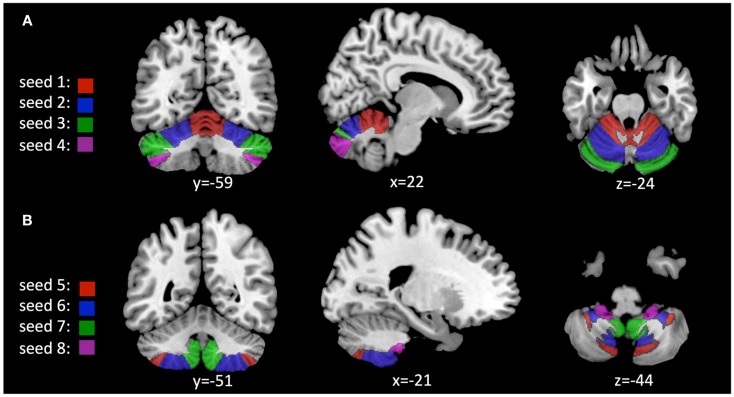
Location of seeds used for analysis of cerebro-cerebellar coupling as derived from the AAL 3D MRI atlas (Schmahmann et al., 1999; Tzourio-Mazoyer et al., 2002): (A) Seeds #1–4, representing cerebellar lobules I–V; VI; crus 1; crus 2. (B) Seeds #5–8, representing lobules VIIb; VIII; IX; X.
